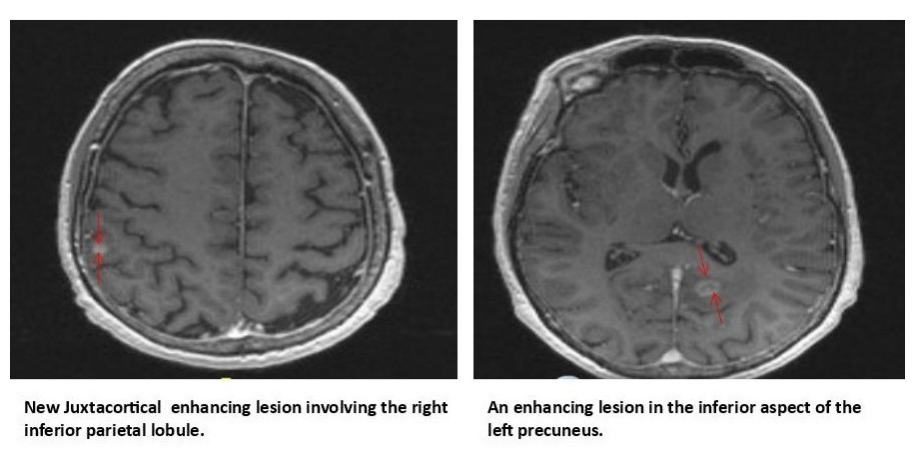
MRI brain with new metastases highlighted by arrows. MRI: Magnetic resonance imaging.
